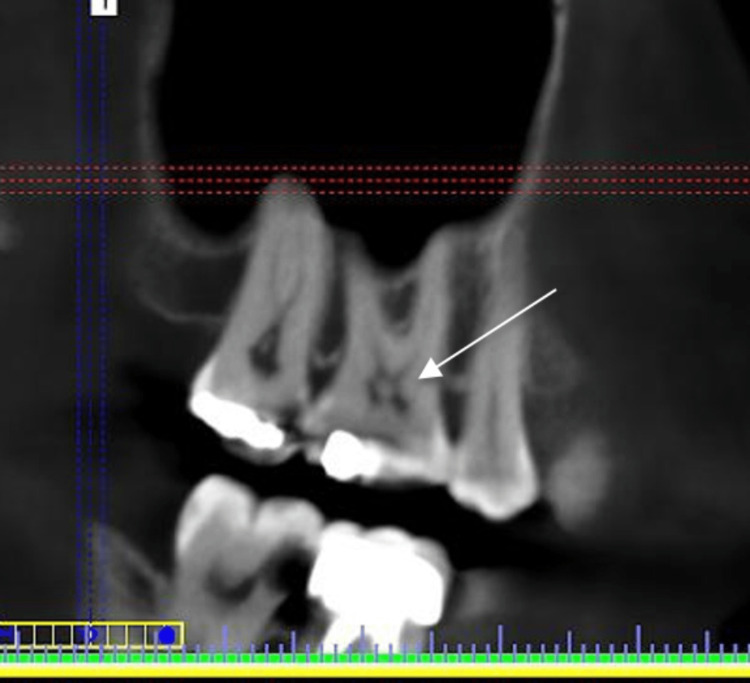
CBCT sagittal section showing pulp stone in the upper first molar CBCT: cone-beam computed tomography
